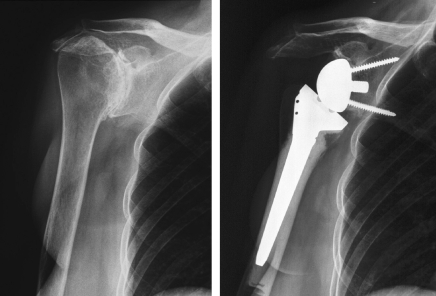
Delta III prosthesis in CTA type V according to Hamada.
